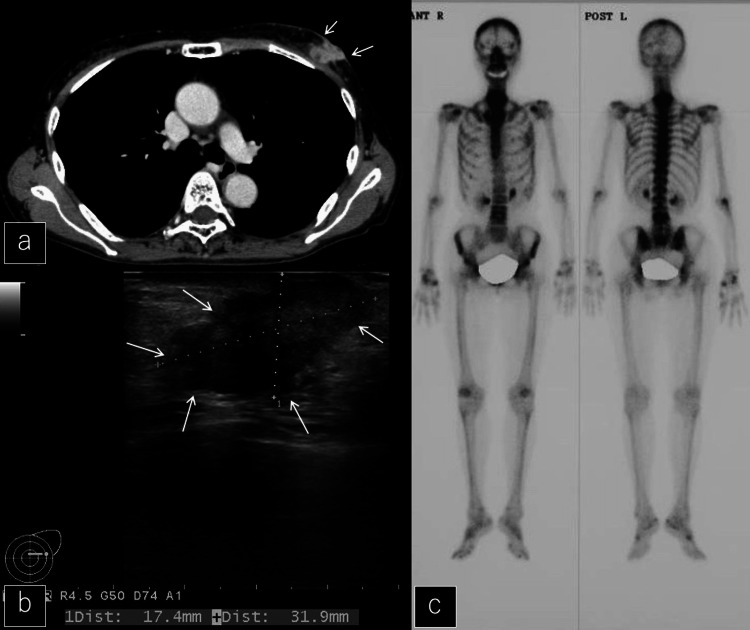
Chest CT, breast ultrasound, and bone scintigram after the first visit a: Chest CT showed an abnormal shadow around a left breast mass (white arrows). b: Ultrasound showed a left mammary tumor (white arrows). c: Bone scintigrams (99mTc) showed multiple bone metastases.
